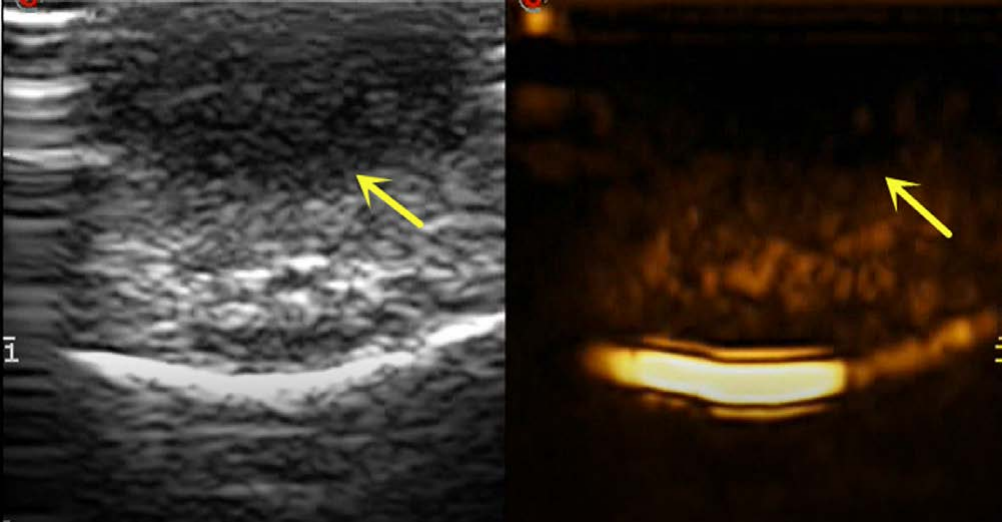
CEUS shows mass is low enhancement (yellow arrow). CEUS = contrast-enhanced ultrasound.
